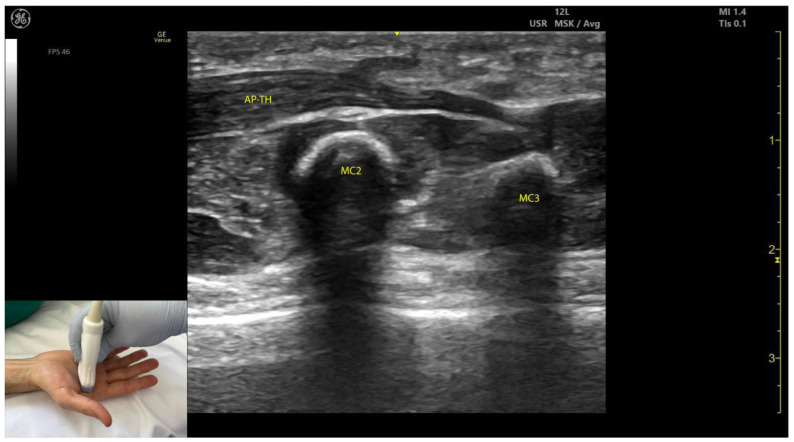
Ultrasound anatomy of the adductor pollicis transverse head (AP-TH) with key landmarks: AP-TH adductor pollicis transverse head; MC2—second metacarpal; MC3—third metacarpal.
